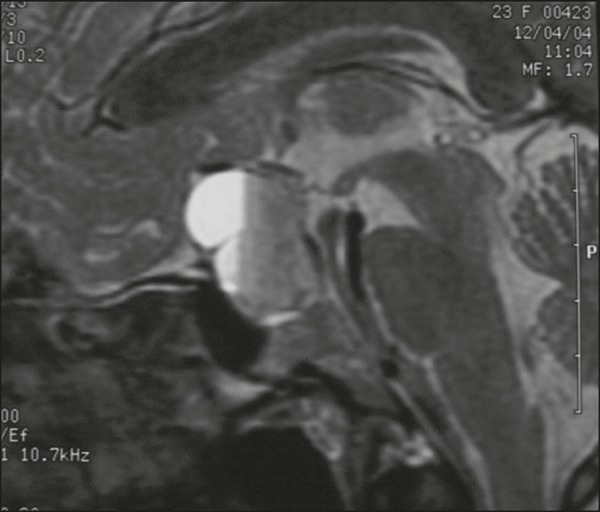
Macroadenoma with pituitary apoplexy. Sagittal T2-weighted MRI sequence
